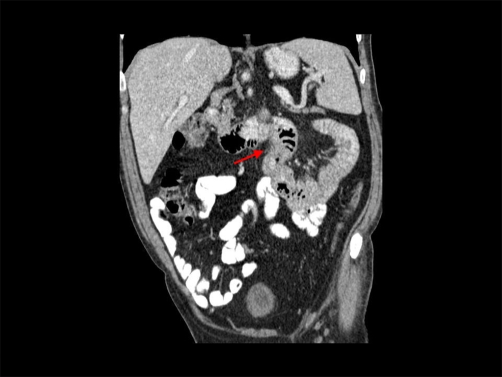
Diagnostic CT scan view showing diffuse upper jejunal thickening.
